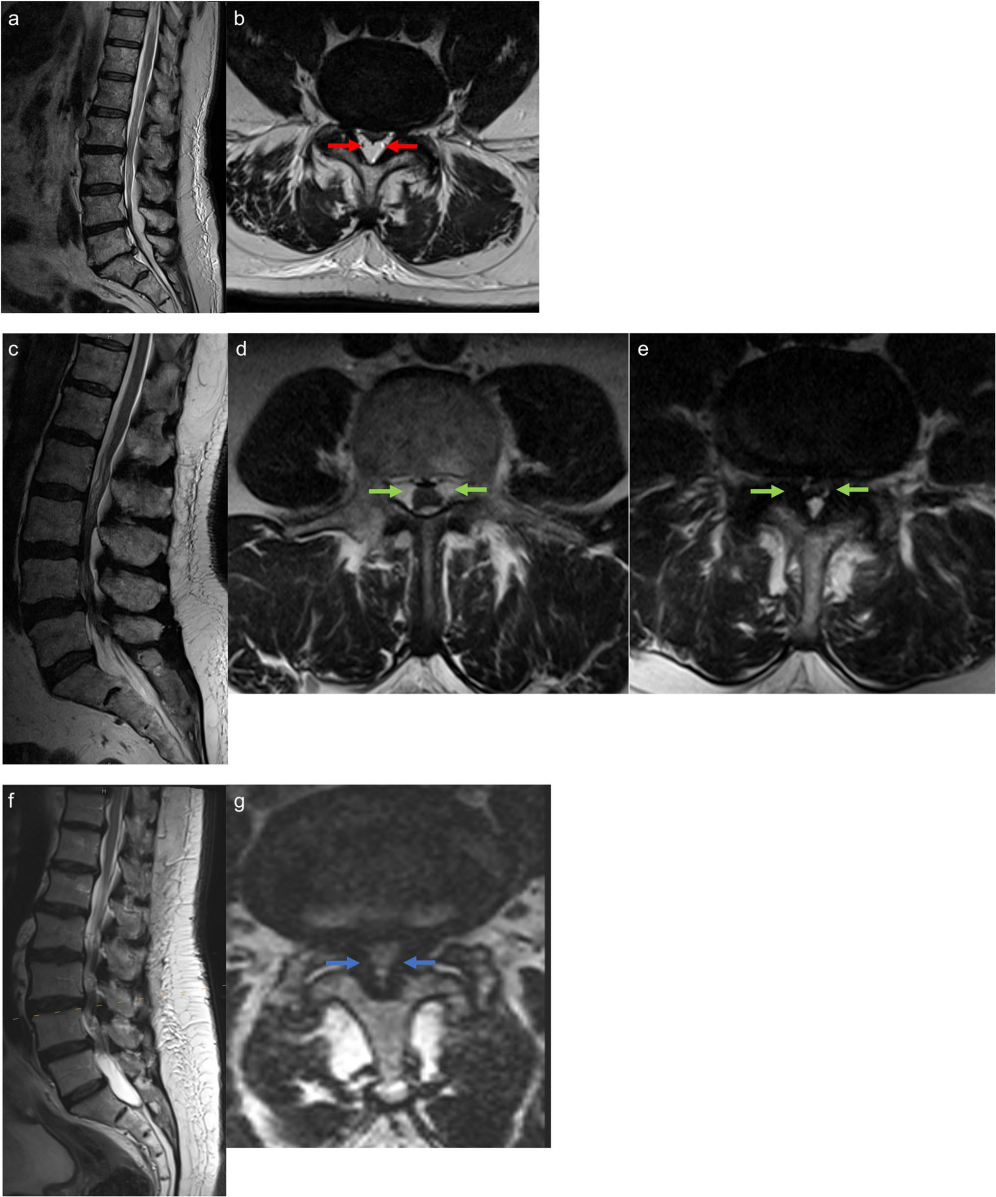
MR imaging (sagittal and axial) of patients with SEL (a, b), SEL+LSS (c, d, e), and pure LSS (f, g). SEL is characterized by abnormal overgrowth of adipose tissue in the epidural space (red arrows, b). The mixed pathology (SEL+LSS) shows in addition to excessive overgrowth of epidural fat in one segment (green arrows, d) also lumbar spinal stenosis due to hypertrophied ligamenta flava in another segment (green arrows, e), whereas in pure LSS, only ligamenta flava thickening is detectable (blue arrows, g)
